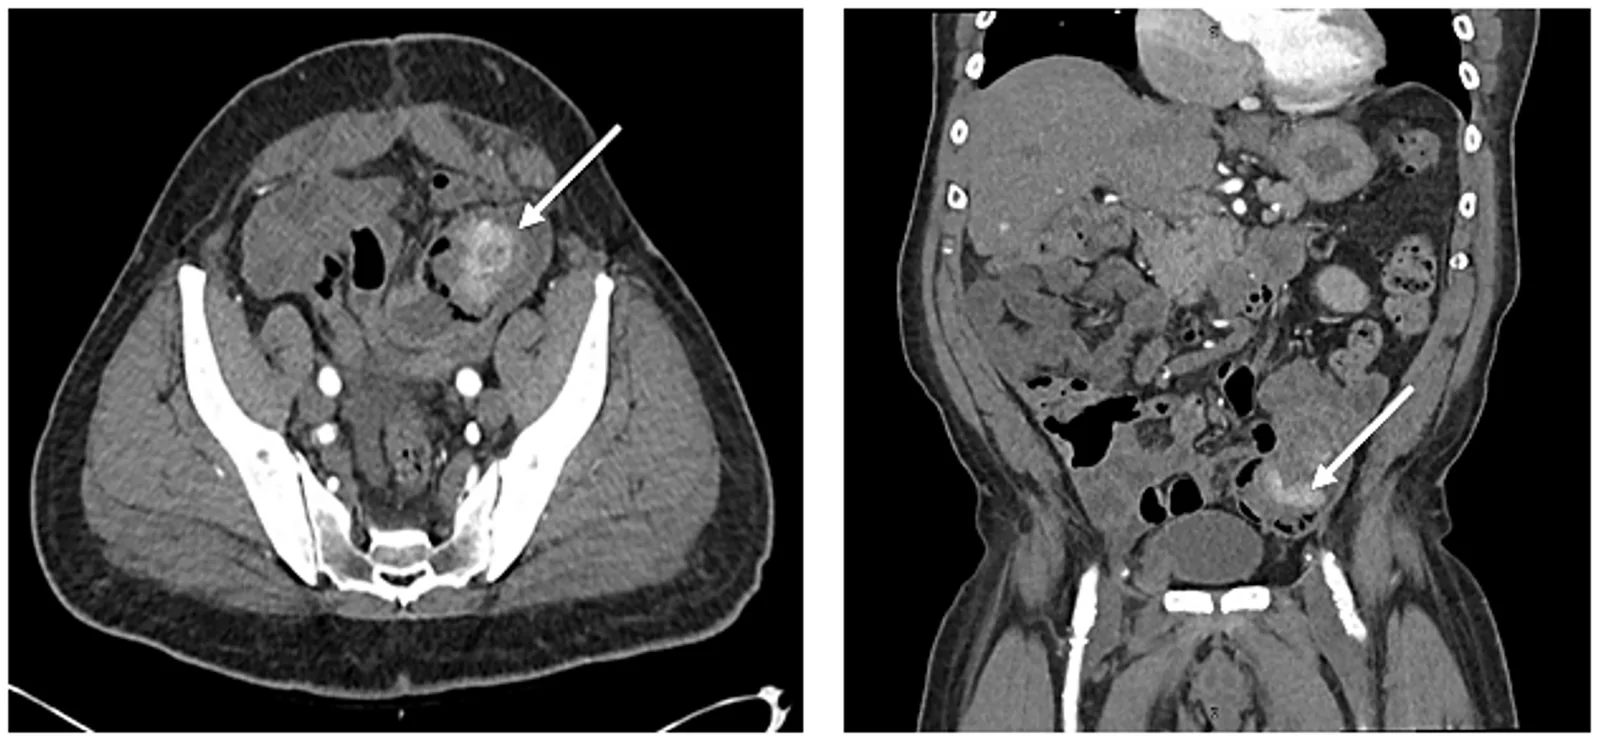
CTA abdomen and pelvis: Axial and coronal views showed an area of active extravasation of contrast in the left lower quadrant (arrows).
